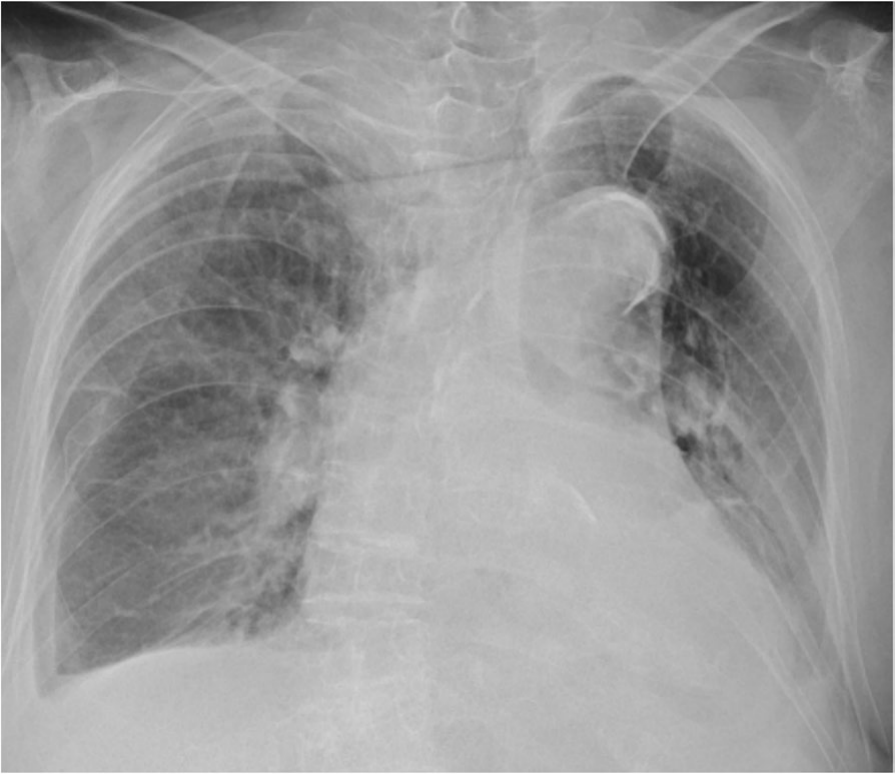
Chest X-ray image showing a mild right-sided pleural effusion
